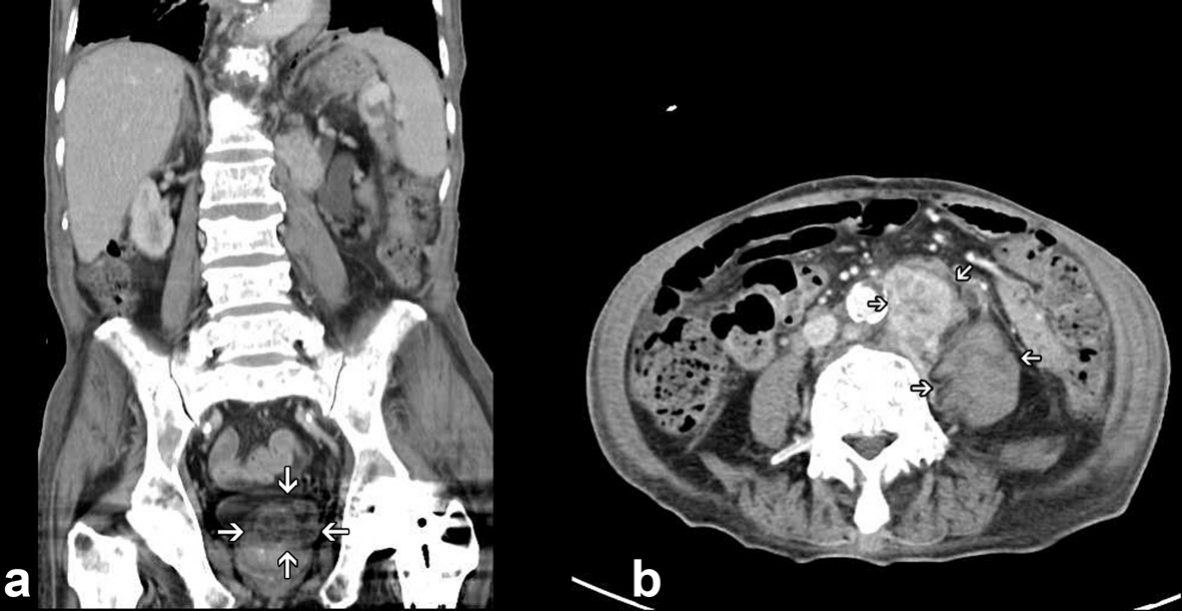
Enhanced lower abdomen CT showing a 4.3 × 3.0 cm ill defined heterogenous mass lesion over left lobe of prostate (a); with extensive para-aortic and retroperitoneal lymphadenopathy (b).
